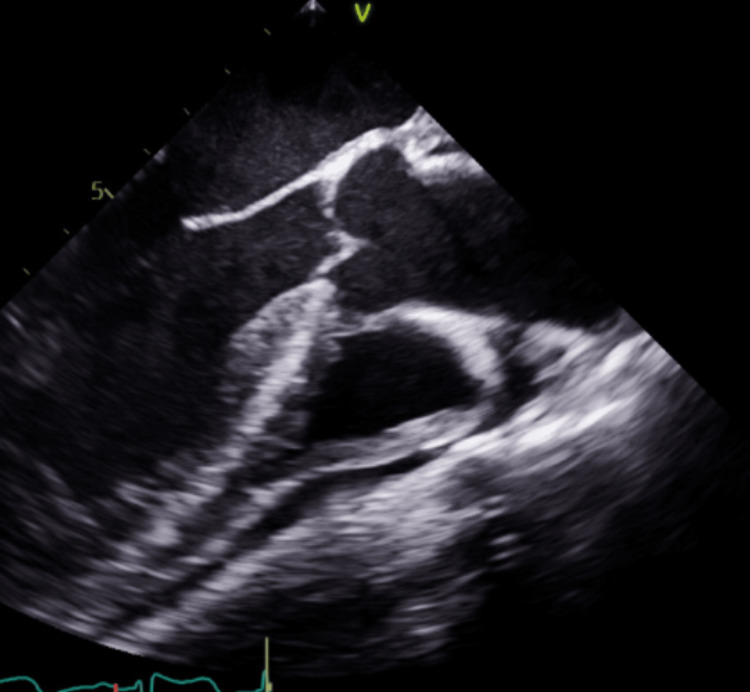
TEE performed 40 days before current TEE. Mid-esophageal long-axis view showing no vegetation on aortic valve. TEE: transesophageal echocardiogram
